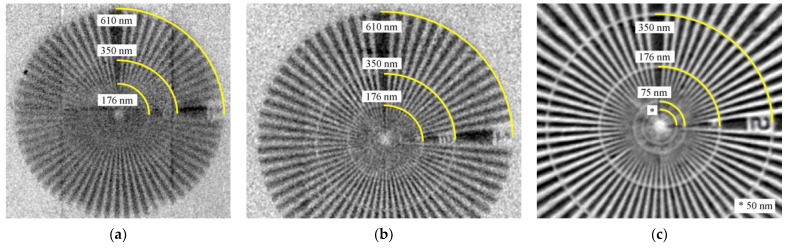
Radiographic images of a Siemens star acquired with a 25° tilted bulk target showing differences in spatial resolution as well as contrast (yellow arcs indicate the defined pattern size. The arc labeled with * corresponds to a pattern size of 50 nm): (a) image acquired with a pixel size of 76 nm using a Cr target; (b) image acquired with a pixel size of 32 nm using a Mo target; (c) image acquired with a pixel size of 45 nm using a Pt target.
